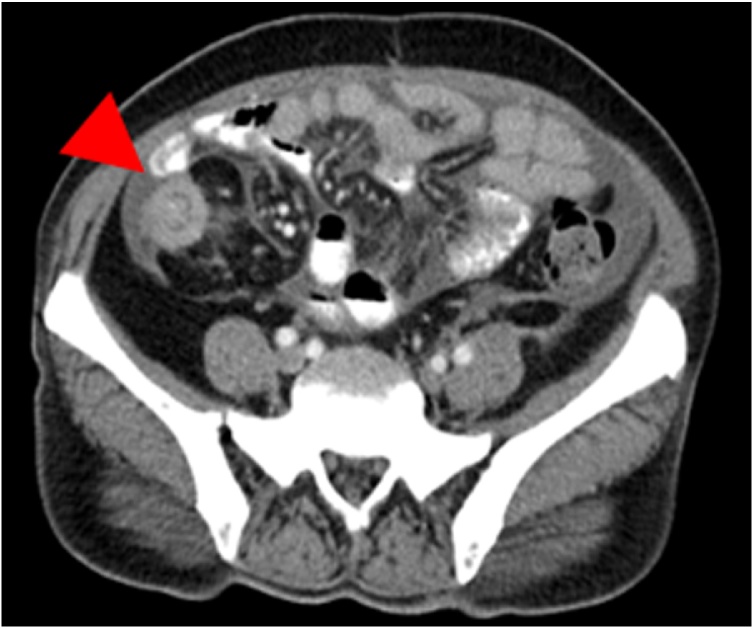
Axial section of the abdominal CT scan demonstrating the target sign of intussusception, marked by a red arrowhead.
